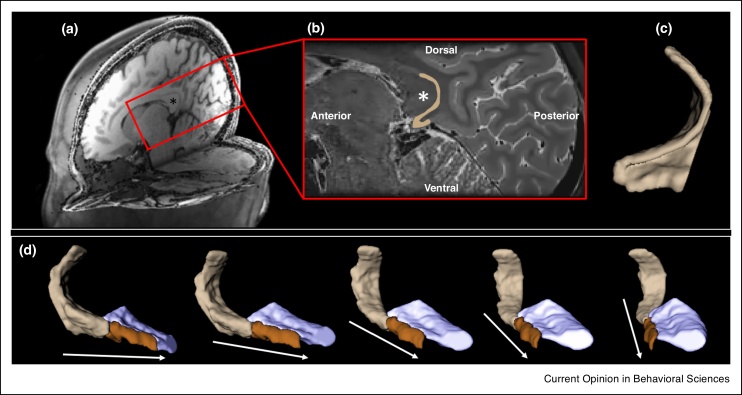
The pre/parasubiculum-retrosplenial cortex continuum. (a) A T1-weighted structural MRI scan presented in 3D with the left hemisphere removed to reveal a medial portion of the right hemisphere. (b) In this T2-weighted structural MRI scan sagittal section, the region encompassed within the red square in (a) is magnified. Note the thin band of the retrosplenial cortex (highlighted beige) hugging the ventral and posterior portion of the corpus callosum [* in both (a) and (b)]. (c) A 3D model of the retrosplenial cortex presented in the same orientation as the sagittal section presented in (b). (d) A 3D model of the retrosplenial cortex (beige), pre/parasubiculum (brown) and the posterior hippocampus (mauve) inclusive of all other hippocampal subregions. On the far left, the model is viewed from a medial perspective. Each consecutive image to the right represents an incremental rotation in a clockwise direction. Arrows indicate anterior. Note the contiguity between the posterior portion of the pre/parasubiculum and anterior portion of the retrosplenial cortex.
